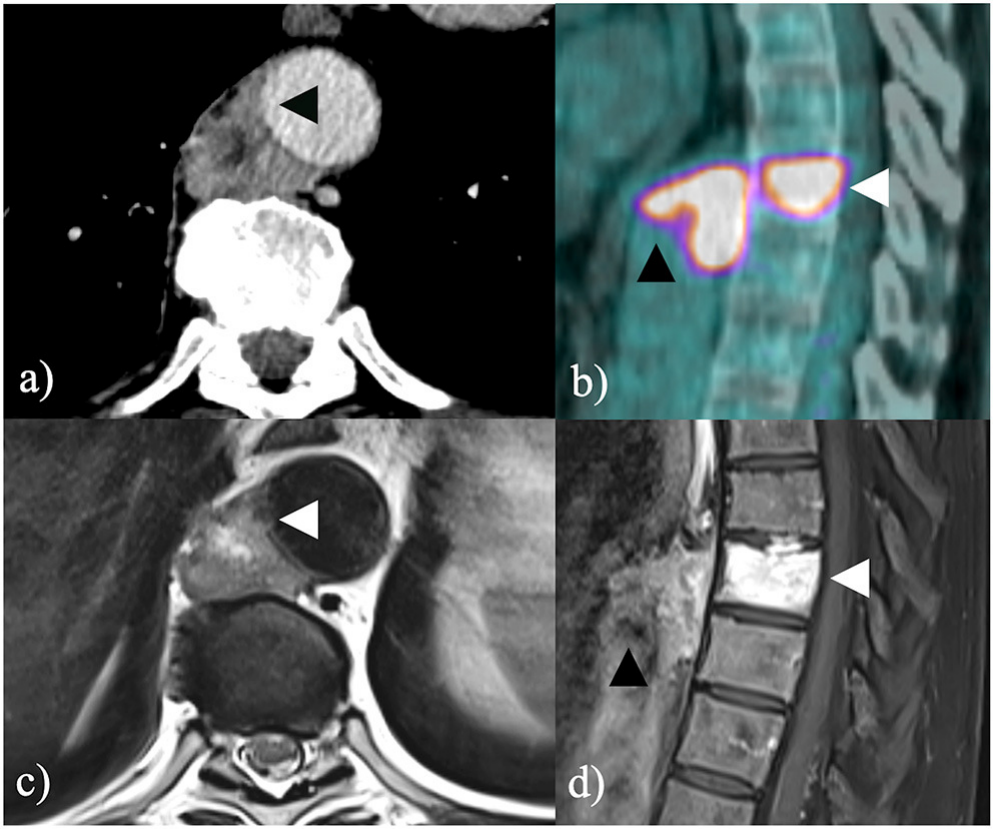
(a) Contrast-enhanced CT scan shows a paravertebral soft tissue mass encasing the thoracic descending aorta with suspicious infiltration of the aortic wall (black arrowhead); (b) further ^18^F-FDG PET/CT shows intense radiopharmaceutical uptake both within the soft tissue tumor (black arrowhead) and within the 9th thoracic vertebra (white arrowhead); (c) contrast-enhanced MRI T1-weighted image confirms infiltration of the aortic wall (white arrowhead) and (d) heterogeneous contrast enhancement both within the soft tissue tumor (black arrowhead) and within the 9th thoracic vertebra (white arrowhead).
